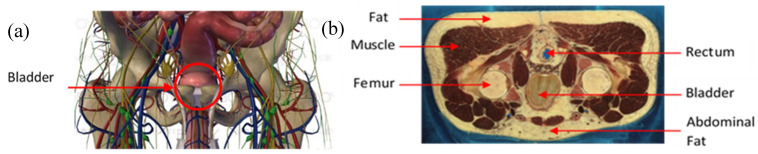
(a) Anatomy of the pelvic cavity showing the bladder’s location; (b) axial computer tomography (CT) scan of the pelvic cavity presenting the nature and location of the tissues.
